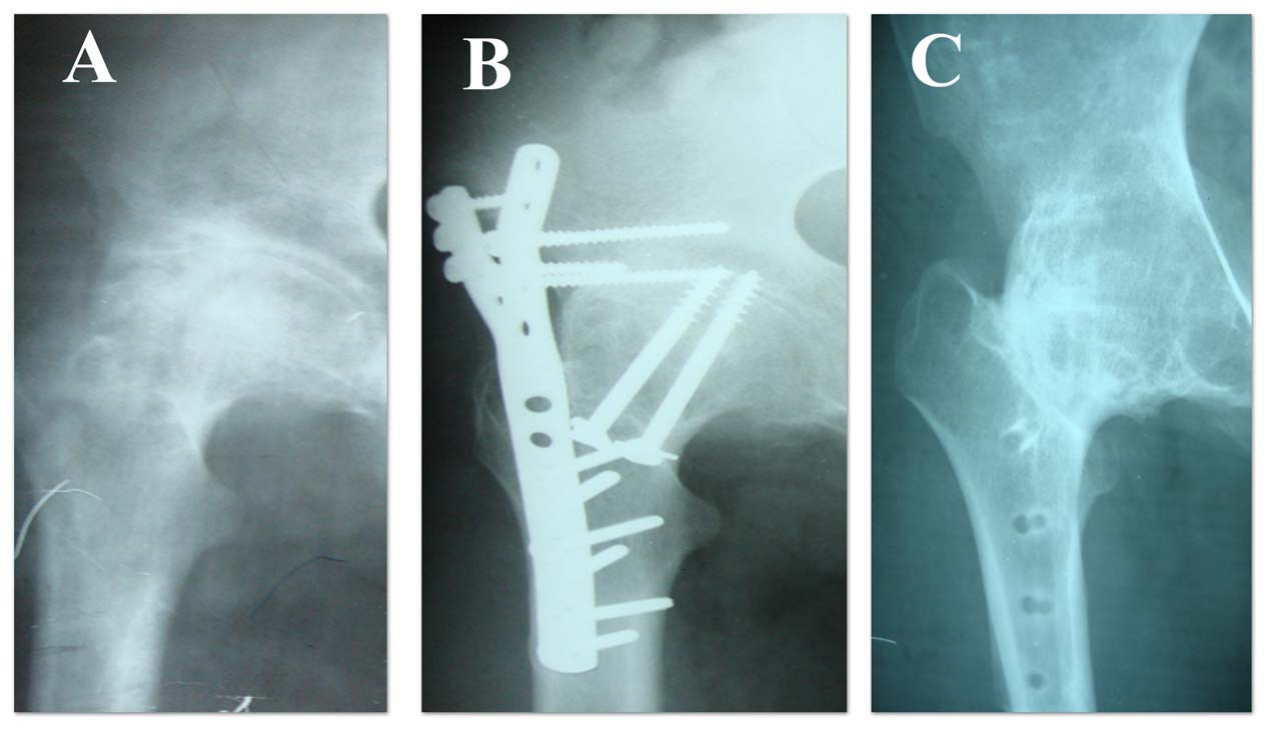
Radiological course of a hip fusion case with the view the anterolateral arthrodesis plate. Radiographs of the right hip in a 35 years old man showing a) a Kellgren 4 hip arthritis secondary to a neglected slipped capital epiphysis, b) the HA with the anterolateral plate 3 months post-operatively, c) fusion of the hip joint and removal of the plate 25 months post-operatively.
